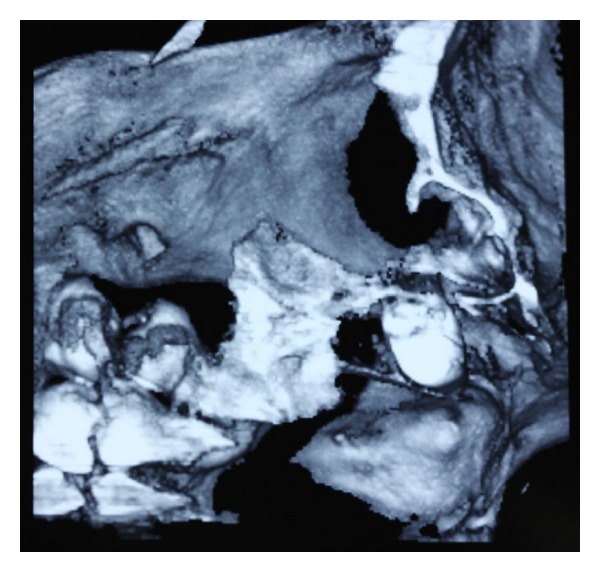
CT revealed that the root was stuck in the antrum of pterygopalatine fossa.
